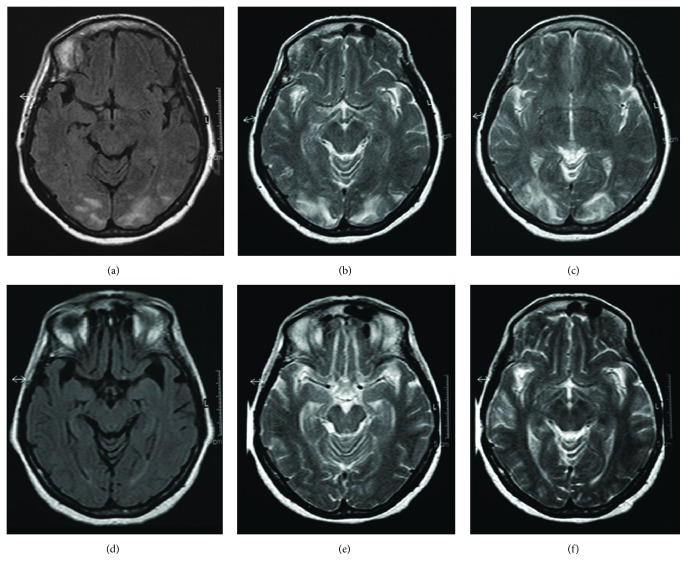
(a, b, c) MRI at the clinical onset of PRES. FLAIR (a) and T2-weighted (b, c) images indicate symmetric hyperintense abnormalities in the occipital lobes. (d, e, f) MRI four weeks after the clinical onset of PRES. FLAIR (d) and T2-weighted (e, f) images show regression of the occipital abnormalities.
